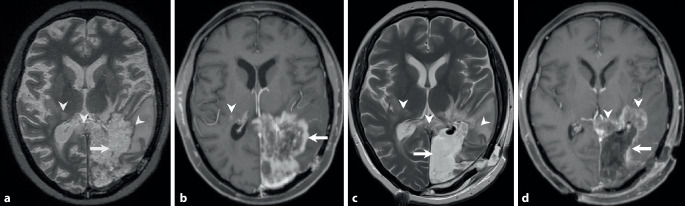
t = 26 weeks. At the end of the second cycle of the intensified chemotherapy with temozolomide the patient complained of mild right-sided hemiparesis. Axial T2-weighted images (a) show an increasing perifocal edema (*arrowheads*) around the tumor resection (*arrow*). On axial T1-weighted images after administration of gadolinium (b) a thick patchy rim of enhancement can be seen along the margins of the initial tumor resection (*arrow*). Note the additional contrast-enhancing lesion located in the white matter adjacent to the right thalamus (*arrowhead*) most likely corresponding to additional manifestation of the underlying tumor. After reoperation a large resection defect can be seen on axial T2-weighted images (c, *arrow*). The perifocal edema is basically stable (c, *arrowheads*). On axial contrast-enhanced T1-weighted images (d) discrete enhancement is visualized along the borders of the tumor resection (*arrow*). Note the bulky contrast-enhancing portions infiltrating the occipital forceps of the corpus callosum and the white matter lateral to the left thalamus (*arrowheads*)
